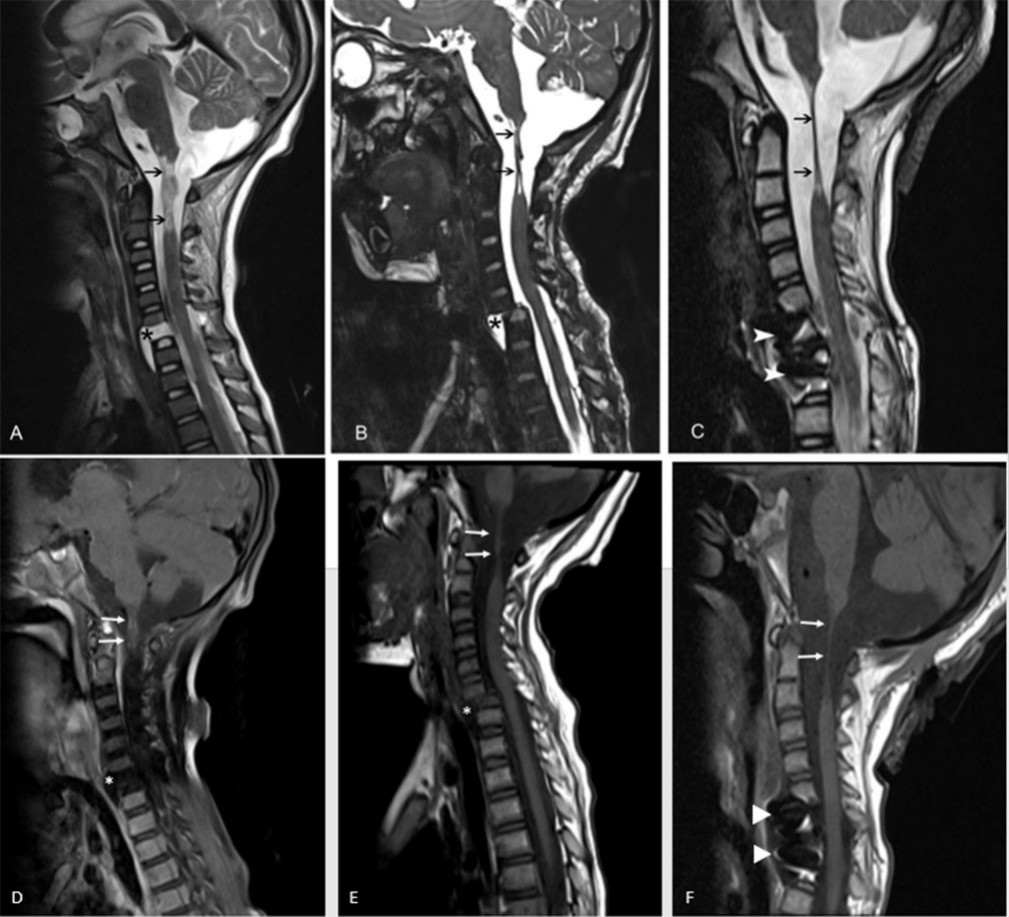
A four-year-old boy who had been involved in a motor vehicle accident was admitted to our pediatric intensive care unit (PICU). During the accident, he was sitting in the child seat in the back of the car, with his seatbelt fastened. The accident occurred when the car was hit by another car when waiting at a red light. Cardiopulmonary resuscitation was applied to the boy for 10 minutes by the rescuers at the site of the accident. In the PICU, he was intubated carefully and mechanically ventilated, and all the other required medical treatments were administered. He was treated in the PICU for a long time. He was discharged with quadriplegia, tracheostomy, and home mechanical ventilator. During the follow-up period, consecutive magnetic resonance imaging (MRI) showed significant and rare findings (Figure 1).
